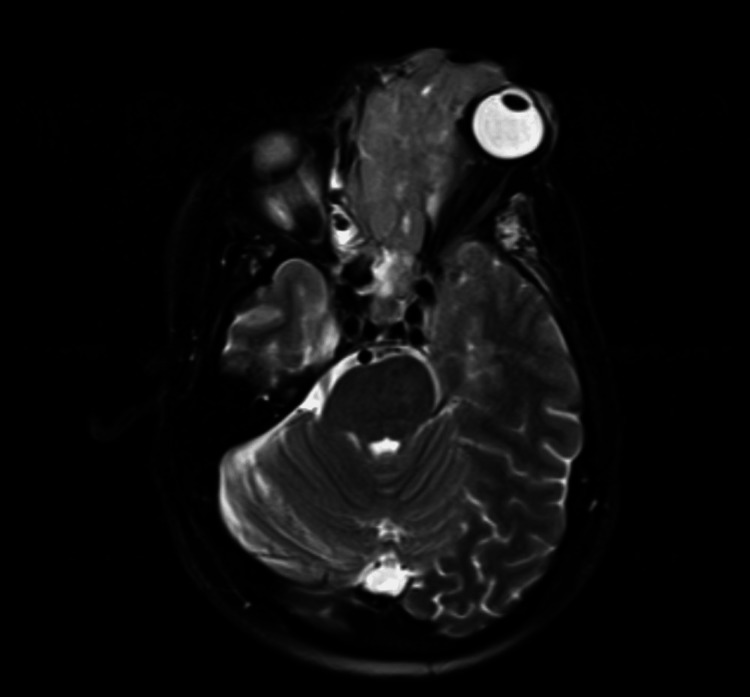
MRI brain with contrast MRI brain, transverse plane of infiltrative mass lesion originating in the orbit measuring with erosion of the walls of the left maxillary sinus, through the left nasal cavity into the bilateral ethmoid air cells, and bilateral sphenoid sinus. This view demonstrates the erosion of the adjacent skull base as well as the left carotid canal and the cribriform plate.
